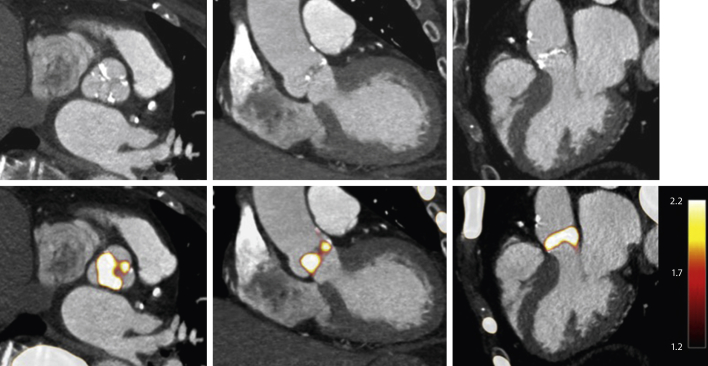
^18^F-NaF PET-CT of the Aortic Valve Contrast-enhanced computed tomography (CT) of the aortic valve (top) with fused radiolabeled sodium fluoride (^18^F-NaF) positron emission tomography (PET)–CT angiography images in the same patient (bottom). Strong PET uptake can be localized to the aortic valve in short-axis (left), coronal (middle), and sagittal (right) views.
